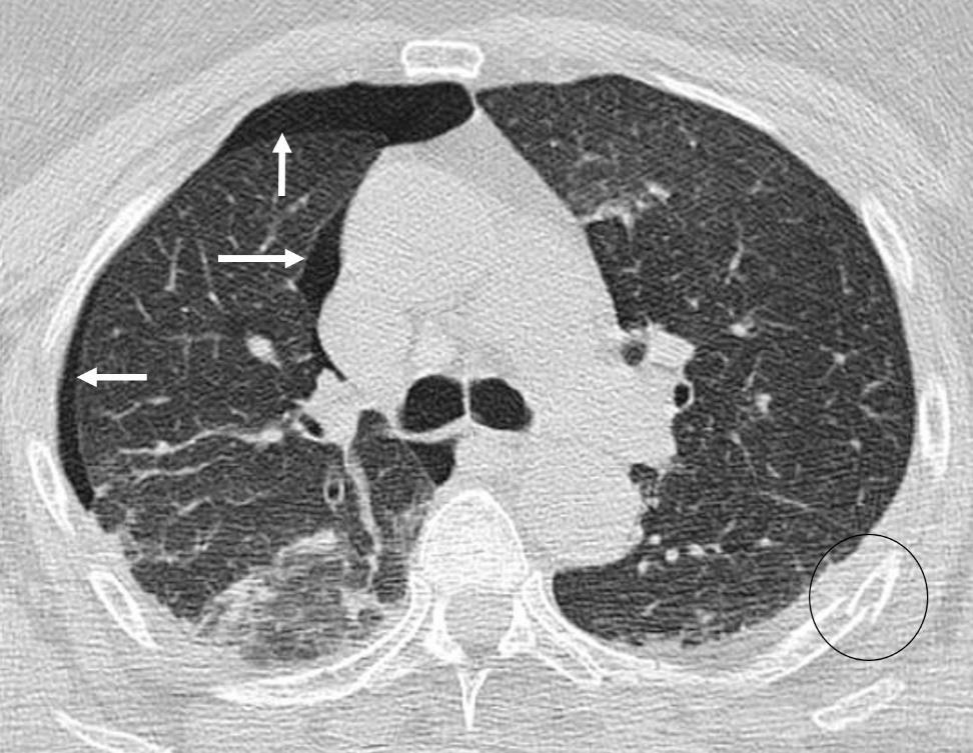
An axial CT image (lung window) of the chest of 21 years old man obtained after a high-speed car crash shows right sided pneumothorax. Solid arrows point to the margin of the displaced visceral pleura. Right oblique fissure is not clearly delineated and is obscured by the ground glass opacities in the posterior aspect of the right lung which given it location is in the right lower lobe and suggestive of pulmonary contusion. Patient had multiple rib fractures bilaterally but only a left sided fracture (circled) is seen on this image. It shall be noted that fractures and other skeletal pathologies are best visible on the bone window
